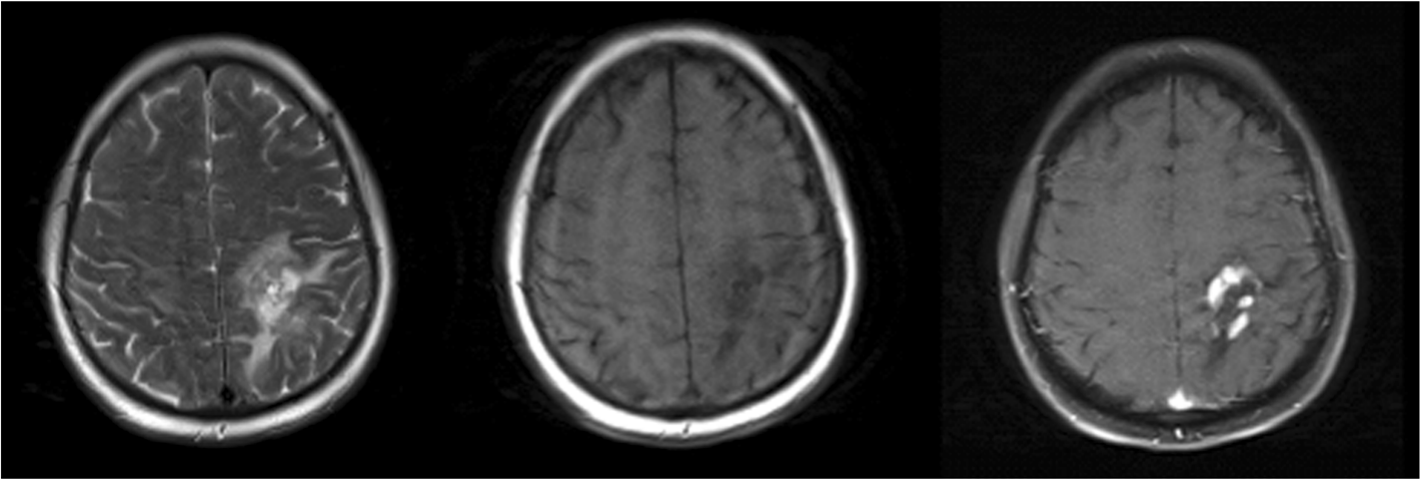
Head MRI enhanced scanning
